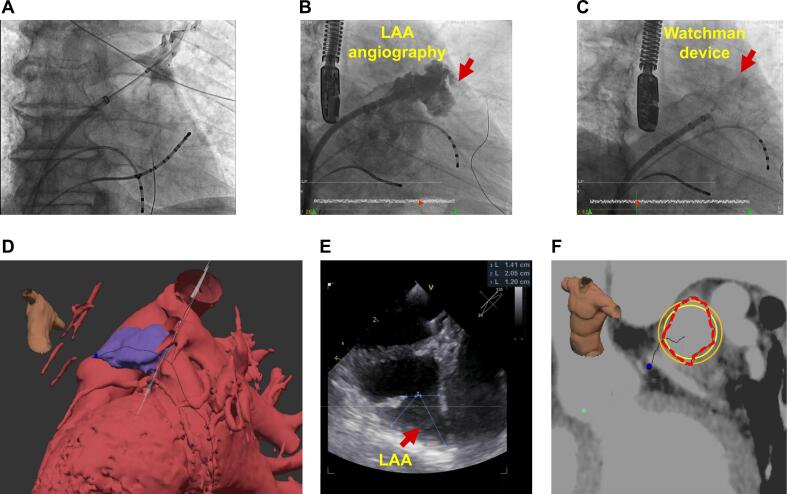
Representative case of cryoballoon ablation with concomitant LAAO In this representative case, we first performed an atrial septal puncture and pulmonary vein isolation (PVi) using a cryoballoon (A), followed by LAA angiography (B), and then implanted a left atrial appendage occluder (C). Preprocedural CT planning images display various angles and automatically highlight the LAA (D). For comparison, an intra-procedural transesophageal echocardiography (TEE) is shown in (E). Analysis of the LAA ostium automatically calculates key anatomical data and provides recommendations for suitable implant size (F).
